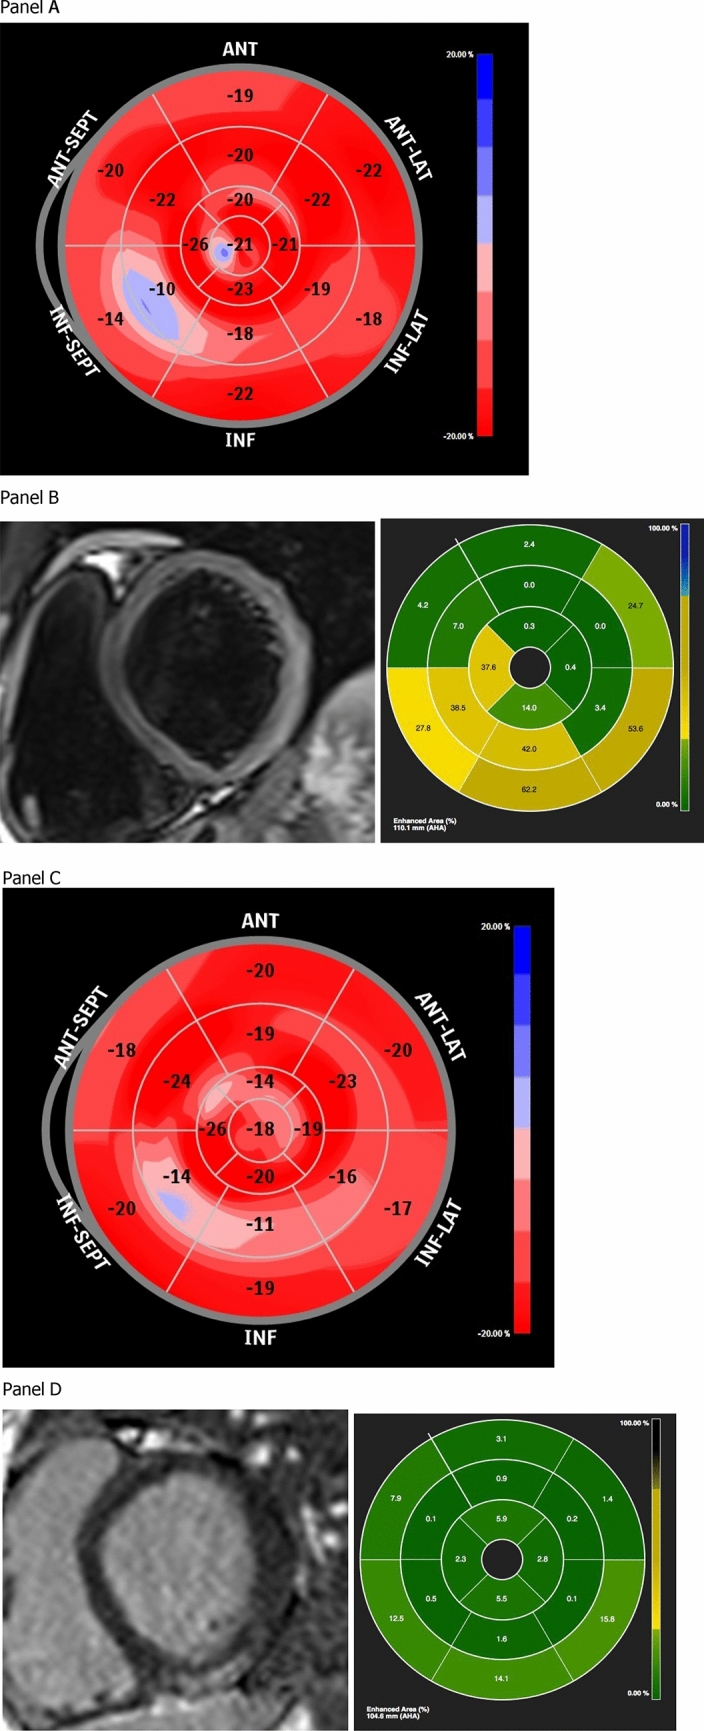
Example of patient with persistent systolic dysfunction at follow-up (Panel A and C images acquired with QLab Cardiac Analysis ver.10, Philips Heathcare Inc., Panel B and D images acquired with CMR42, Circle Cardiovascular Imaging, Calgary, AB, Canada). (A) Evidence of impaired GLS at baseline echocardiographic evaluation. (B) Evidence of significant focal edema at baseline CMR. (C) Evidence of persistent impaired GLS at follow-up echocardiographic evaluation. (D) Evidence of residual focal fibrosis at follow-up CMR.
